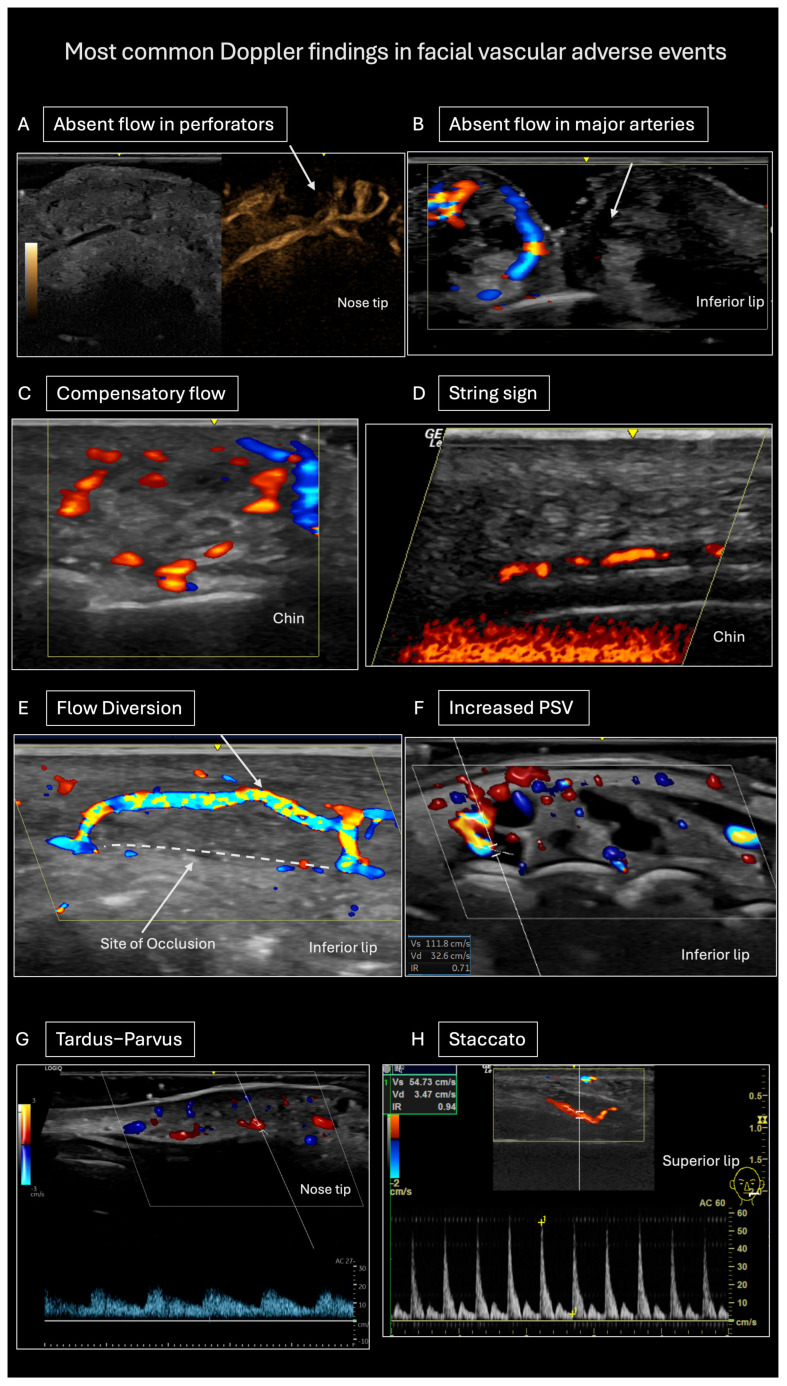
Images of Doppler ultrasound findings in filler-related VAEs. (A) B-Flow US image of a 45-year-old female participant 7 days after hyaluronic acid in the nose showing absent flow of perforators on the tip of the nose. (B) Color Doppler US images showing absent flow in the inferior labial artery in a 25-year-old female participant one hour after the injection of hyaluronic acid in the lower lip. (C) Color Doppler ultrasound images in a 30-year-old male participant, who received a hyaluronic acid injection in the chin 17 h prior to the exam, demonstrate compensatory flow surrounding a region without detectable Doppler signal (silent area). (D) Power Doppler ultrasound of a 55-year-old female participant who received a hyaluronic acid injection in the lower lip 48 h prior to the ultrasound examination shows a string sign (filiform flow) in the right labiomental artery. (E) Color Doppler US in a 22-year-old female injected with hyaluronic acid in the lips demonstrates occlusion of the inferior labial artery, with flow diversion to a branching vessel bypassing the site of occlusion. (F) PW Doppler of the inferior labial artery in a 66-year-old female participant demonstrates increased PSV in the inferior labial artery, which is surrounded by a hyaluronic acid deposit. (G) PW Doppler of a 33-year-old male injected with hyaluronic acid in the nose demonstrating a damped flow pattern (tardus–parvus waveform) in the nasal artery. (H) PW Doppler of a 42-year-old female injected with hyaluronic acid demonstrates a high resistive index in the superior labial artery, corresponding to a staccato waveform.
